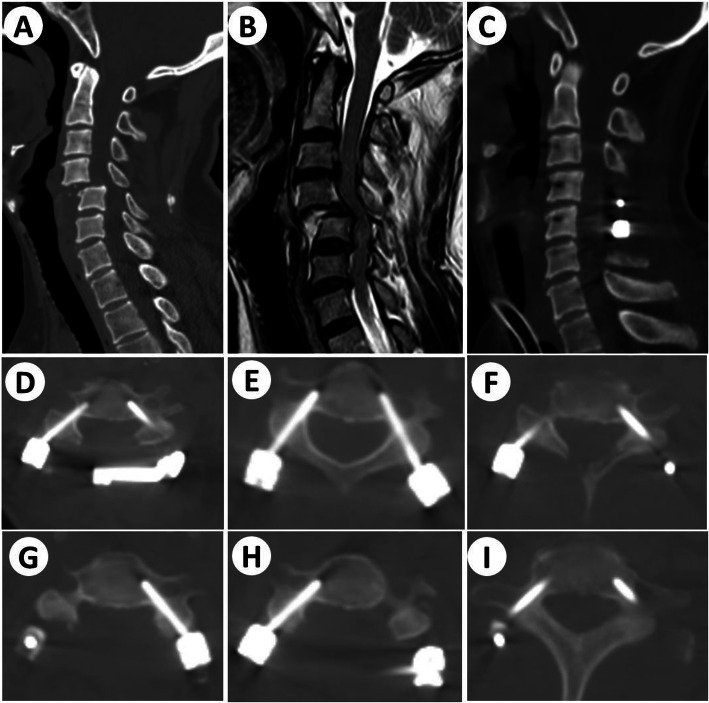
Example of a patient with cervical trauma. a Preoperative cervical CT scan, b Preoperative cervical MR imaging, c Sagittal CT image after cervical operation, d-i transverse CT images after cervical operation
